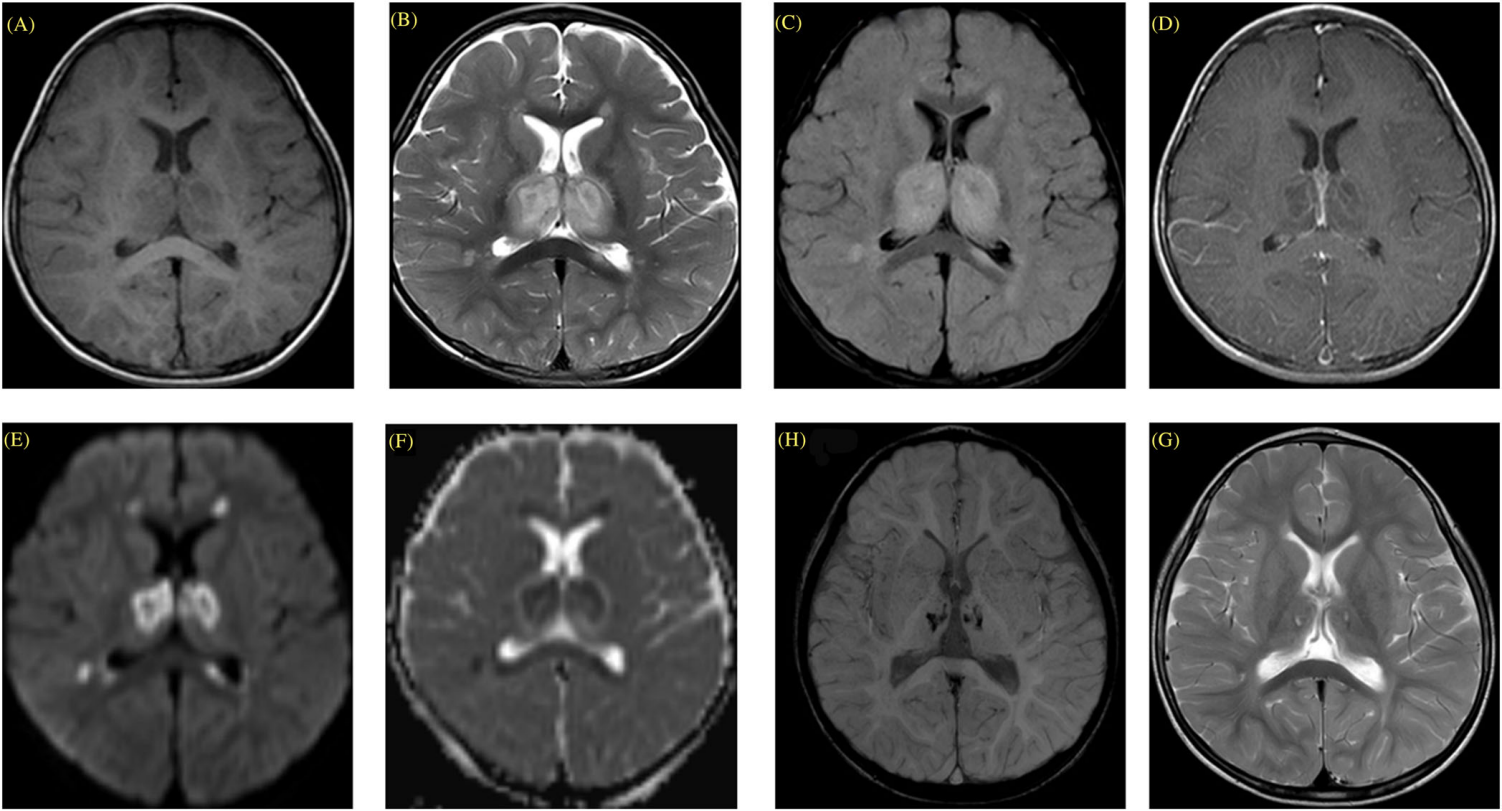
Brain magnetic resonance imaging (MRI) of acute necrotizing encaphalopathy (Case No. 2). (A–F) Initial MRI shows typical brain imaging of acute necrotizing encephalopathy. (A) Axial longitudinal relaxation time (T1)‐weighted imaging (T1WI), (B) transverse relaxation time (T2)‐weighted imaging (T2WI), (C) T2WI/fluid‐attenuated inversion recovery (FLAIR), (D) contrast‐enhanced T1WI, (E) diffusion‐weighted imaging (DWI), and (F) apparent diffusion coefficient (ADC) demonstrate bilateral symmetrical rim‐enhancing thalamic lesions, showing internal T1 hypointensity and T2 hyperintensity with restricted diffusion and central hypointense hemorrhagic foci. Perilesional edema with mild mass effect is seen. These findings correspond with classic target‐like appearance. (G, H) Repeated brain MRI at 7 months after diagnosis, (G) axial T2WI, and (H) susceptibility‐weighted imaging (SWI) show evolutional change of bilateral thalamic lesions to encephalomalacia–gliosis with hemosiderin deposition.
